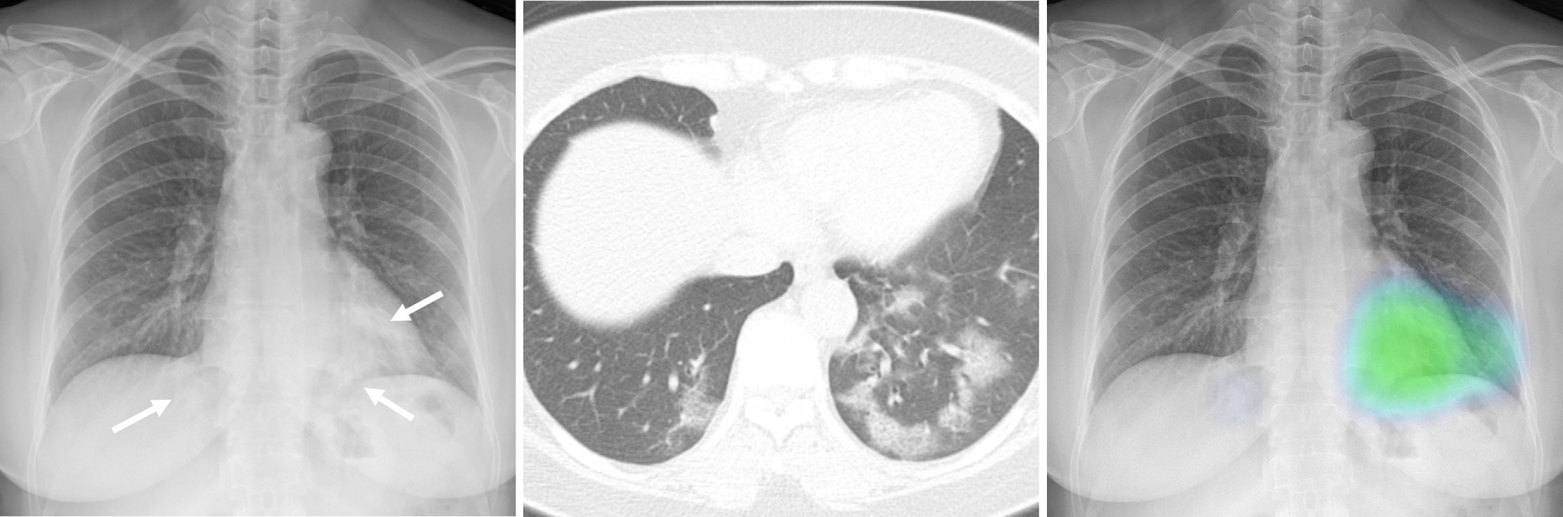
Representative case with COVID-19 pneumonia. A CXR (A) of a patient with confirmed COVID-19 shows patchy infiltrates in both lower lung fields (arrows). The corresponding chest CT image (B) obtained in the same day with the CXR shows multifocal patchy ground-glass opacities in both lower lobes of the lung. The CT severity score of the patient was 13. The CAD system correctly detected pulmonary infiltrates with a probability score of 56% (C). In the reader-alone interpretation, four thoracic radiologists correctly identified the abnormality while none of the non-radiologist physicians identified the abnormality. In the CAD-assisted interpretation, all five thoracic radiologists and four non-radiologist physicians identified the abnormality.
